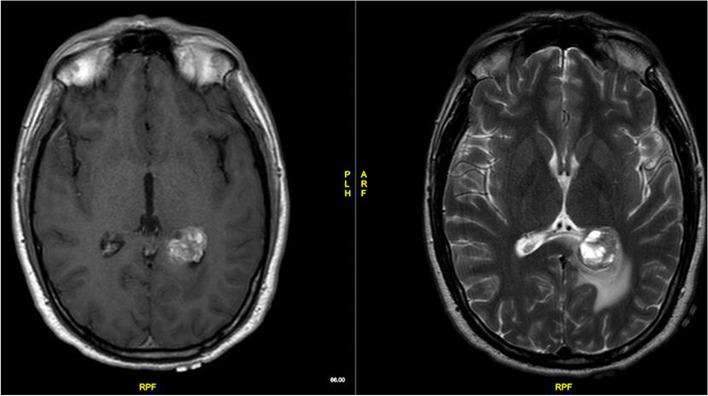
Pre-operative T1 post gadolinium contrast and T2 axial MRI in 2013. There is a 22 mm x 32 mm contrast enhancing circumscribed mass at the atrium of the left lateral ventricle surrounded by oedematous adjacent cerebral parenchyma. The lesion shows mixed solid and cystic components. It appears related to the local choroid plexus. Mild linear enhancement of the ependymal surface of the left occipital horn is suggestive for local infiltration outside of the lesion
